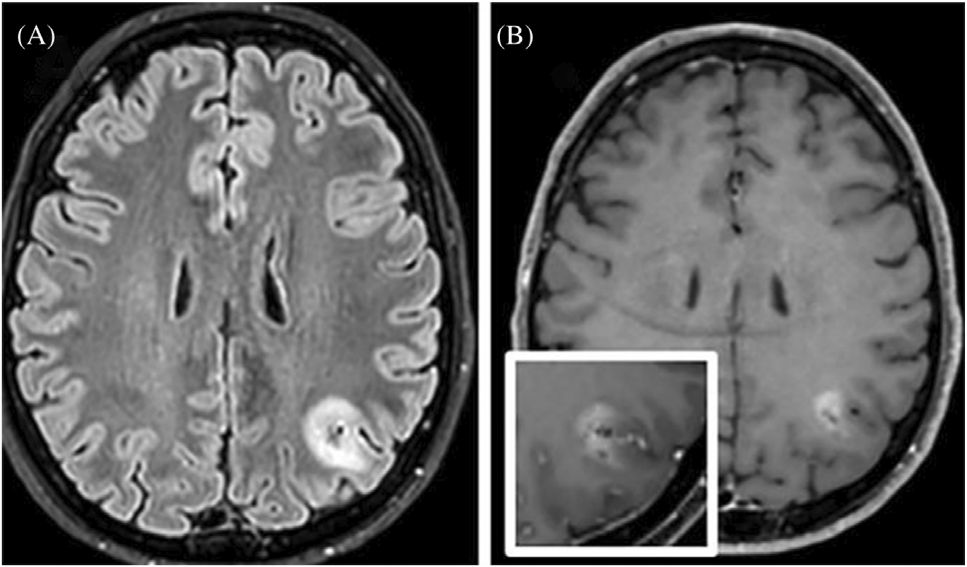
Magnetic resonance imaging showing hyperintensity on FLAIR (A) and heterogeneous hyperintensity on T1 (B) with focal nodular gadolinium contrast enhancement (inset).
